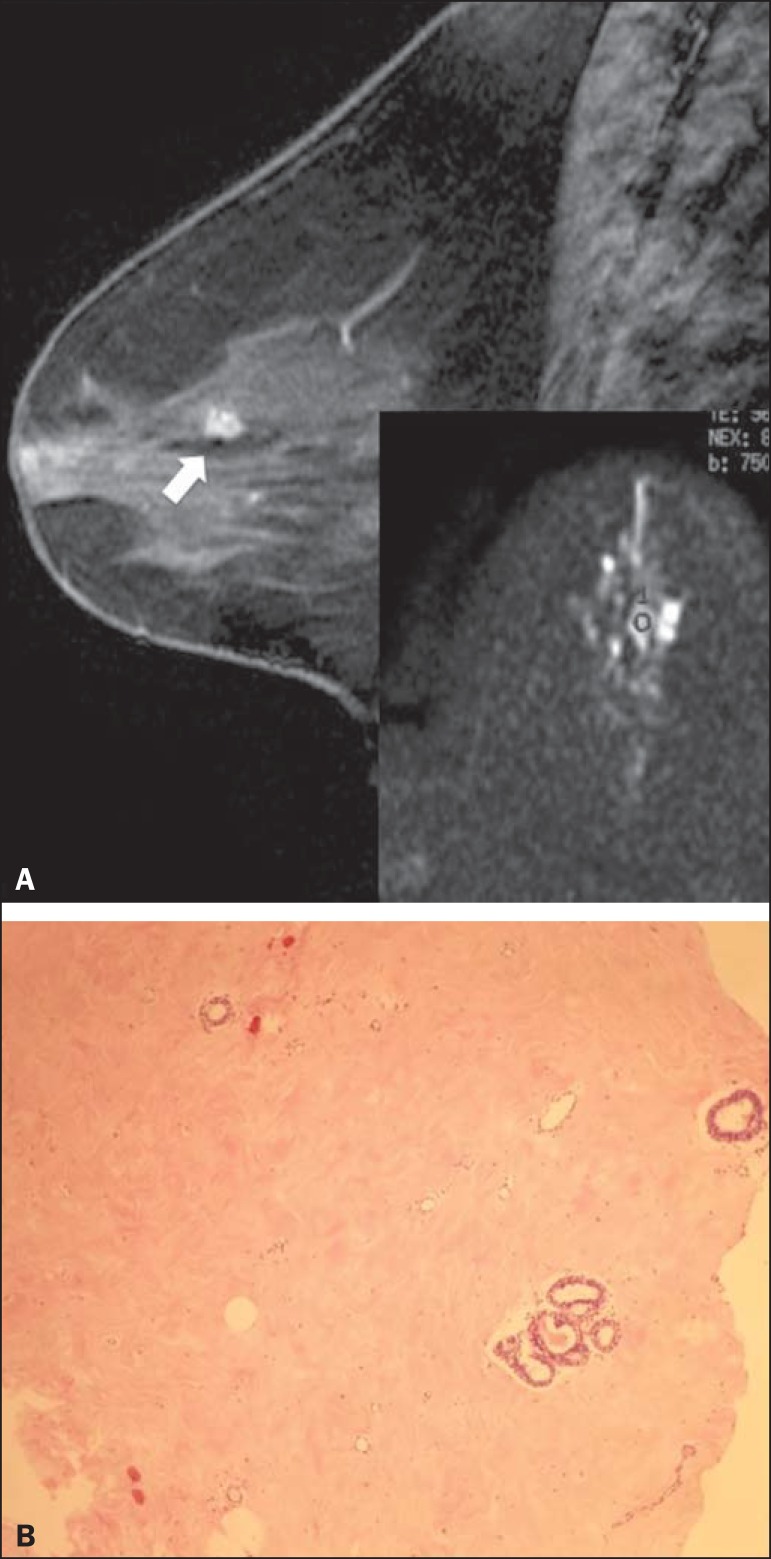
Contrast-enhanced sagittal T1-weighted fat-saturated MRI scan
